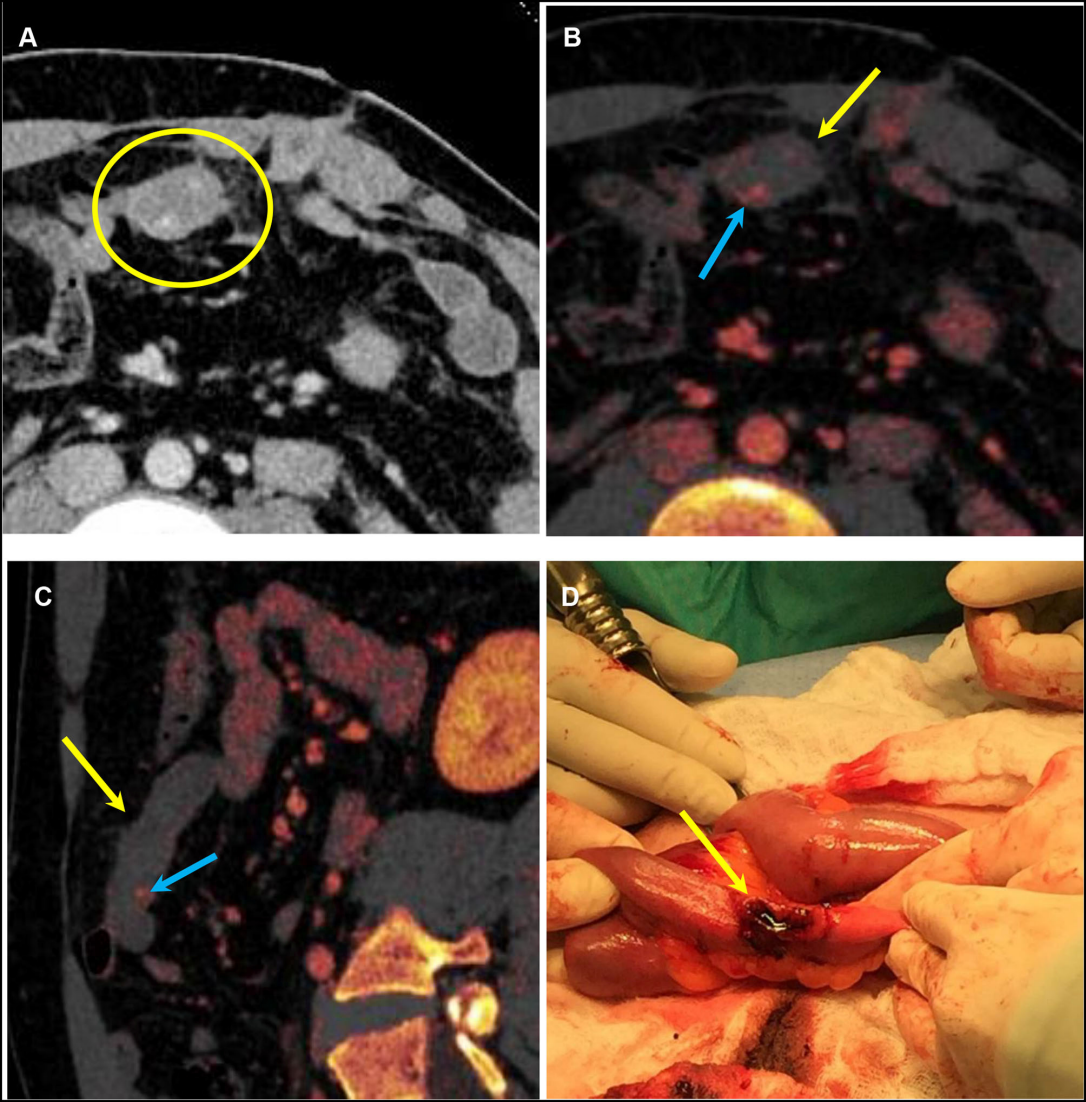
A 25-year-old male patient with a stab wound to the anterior abdomen. (A) Conventional mixed DECT image demonstrates mild thickening of a small bowel loop (yellow arrow). [(B) Axial and (C)] Sagittal oblique DECT iodine overlay images demonstrating an area of transmural lack of iodine uptake anteriorly (yellow arrows) and a small area of endoluminal contrast extravasation not seen on the conventional image (blue arrows) concerning a penetrating injury. (D) Intraoperative picture confirming the focal transmural small bowel perforation (yellow arrow). DECT, dual-energy CT.
